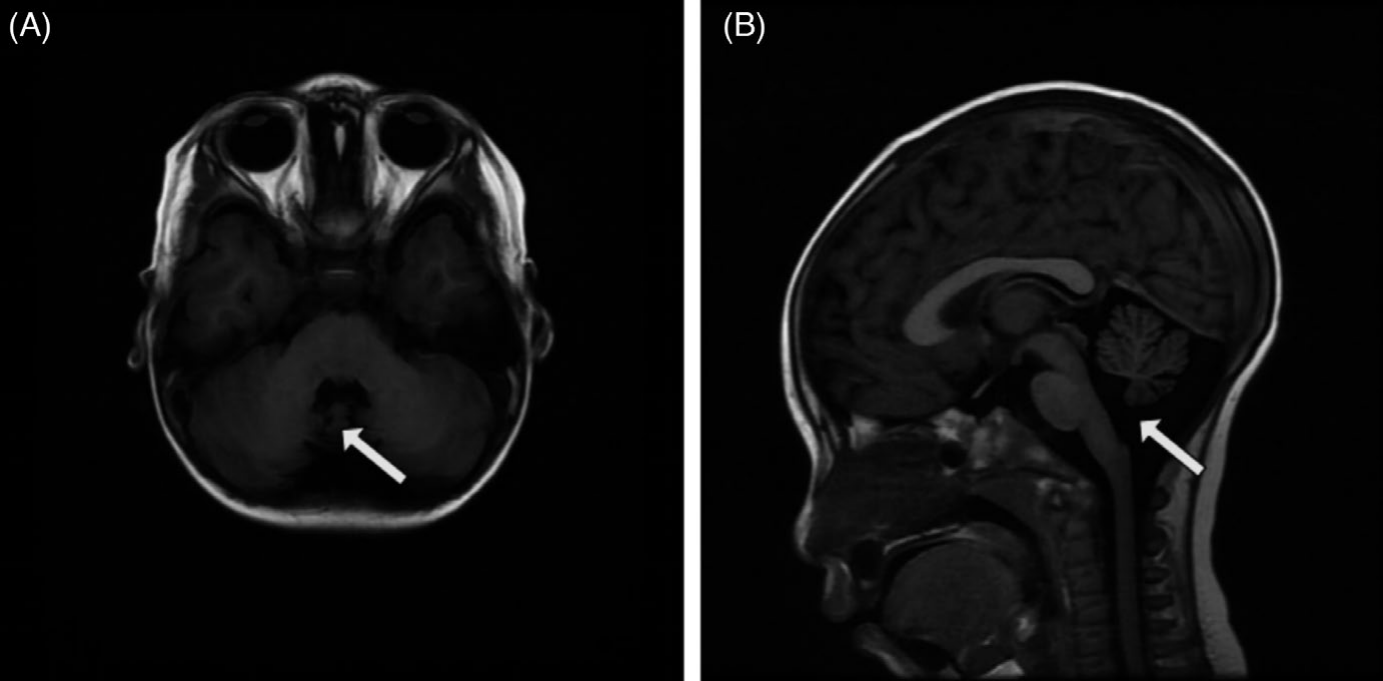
Brain MRI findings. (A) Axial T1‐weighted image showing dysplasia of inferior cerebellar vermis. (B) Mid‐sagittal T1‐weighted image showing cerebellar atrophy mainly in the vermis, and the fourth ventricle enlarged
